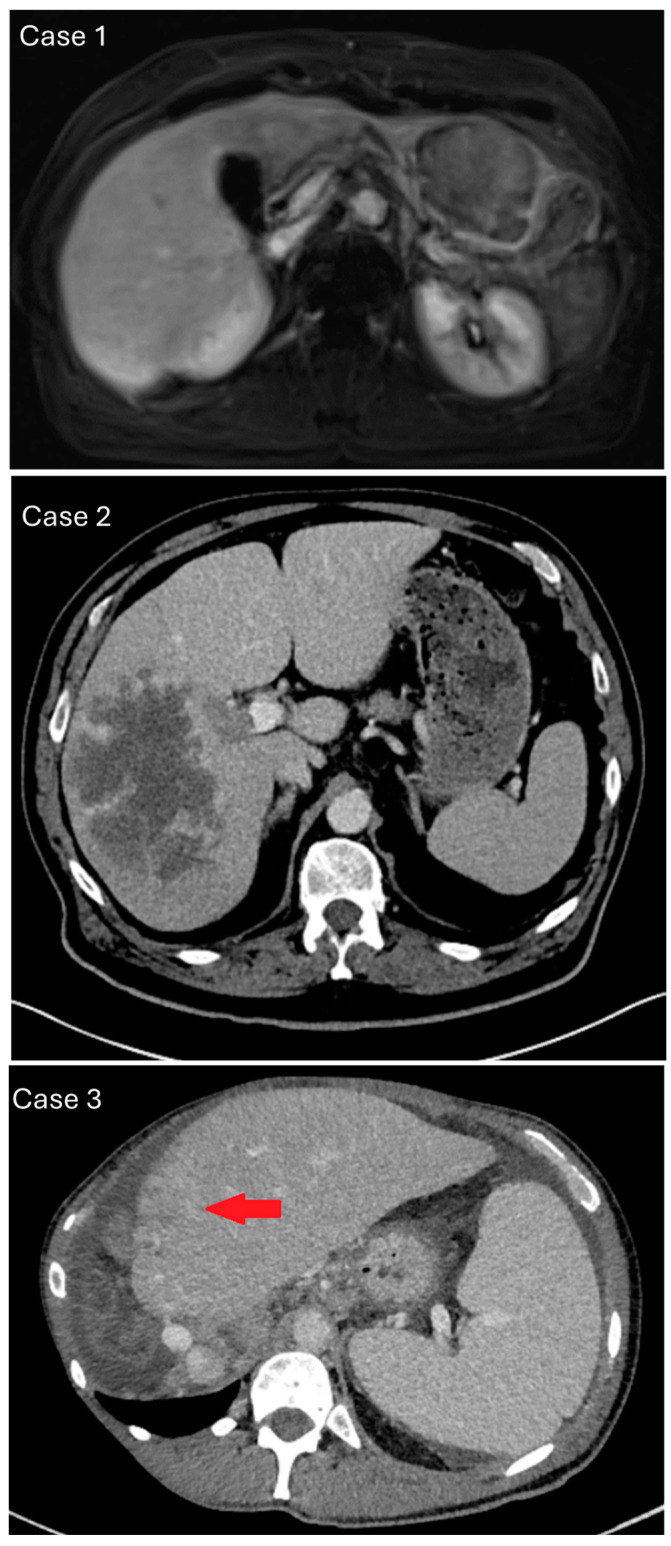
Representative chronological imaging of the case series. Upper panel/case 1: contrast-enhanced magnetic resonance imaging, arterial rim enhancement of approx. 6 cm large, suspicious liver lesion in segment II/III without CCA-typical capsular retraction. Middle panel/case 2: contrast-enhanced computed tomography, venous phase with cauliflower-like mass of right liver without border or capsule, suggesting intrahepatic cholangiocarcinoma (iCCA). Recognizable macrovascular infiltration, which is more common in hepatocellular carcinoma. Lower panel/case 3: contrast-enhanced computed tomography and a new, rim-enhancing mass in liver segment II with capsular retraction and signs of macrovascular infiltration (red arrow).
